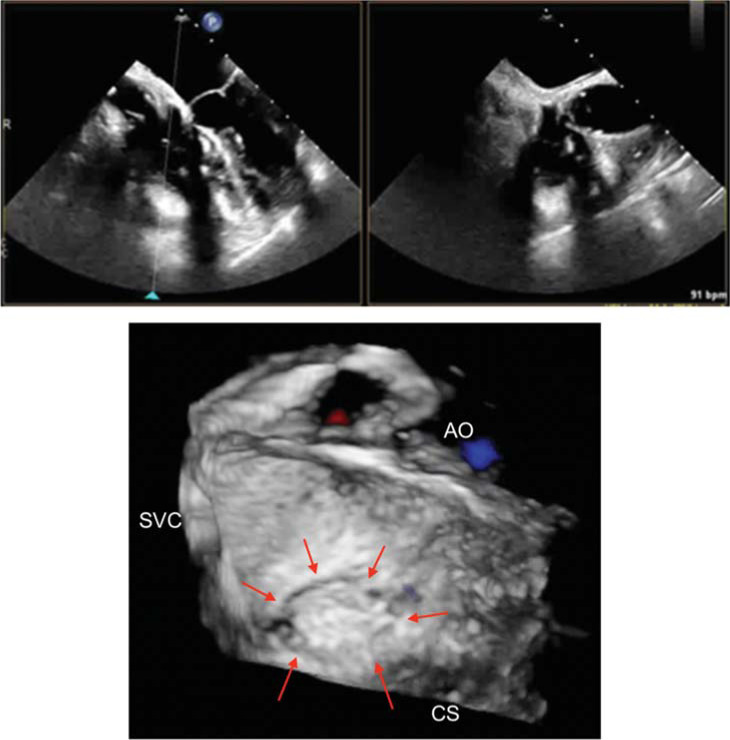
On top, biplane images can be seen. On bottom is a 3-dimensional transthoracic echocardiogram with color flow Doppler of the intact intra-atrial septum (red arrows surrounding the fossa ovalis) without evidence of shunt at the interatrial level. *Abbreviations:* AO, aorta; CS, coronary sinus; SVC, superior vena cava.
